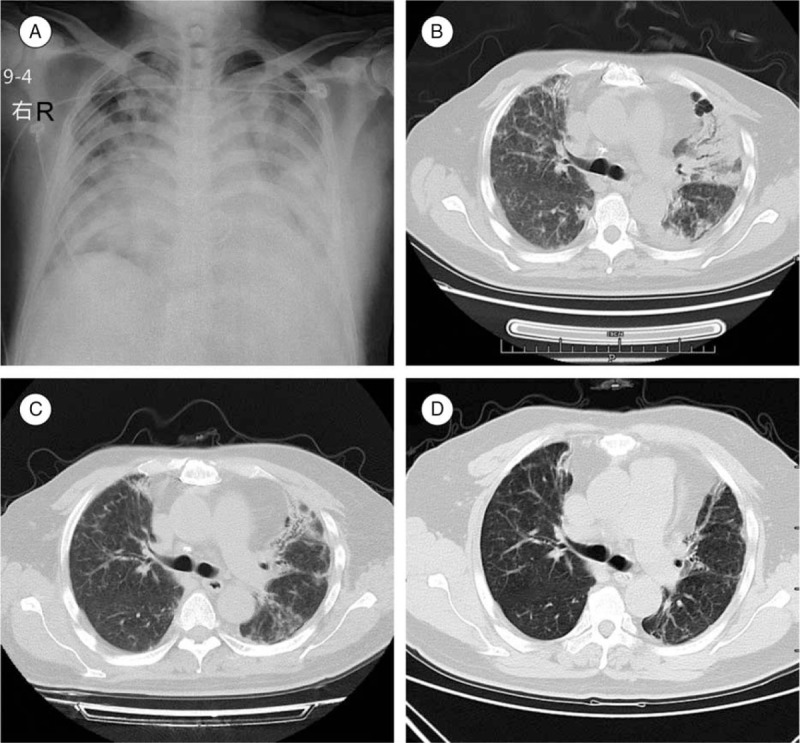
(A) Chest X-ray at admission showed diffuse inflammation and fibrosis of both lungs. (B) CT scan at admission revealed severe lung infection with fibrosis, and the heart in postoperative of mechanical valve replacement changes. (C) CT scan at discharge showed moderate fibrosis and inflammation, improved significantly comparing to CT at admission. (D) 1-year follow-up of chest CT showed multiple chronic infections with fibrosis of both lungs. CT = computed tomography.
